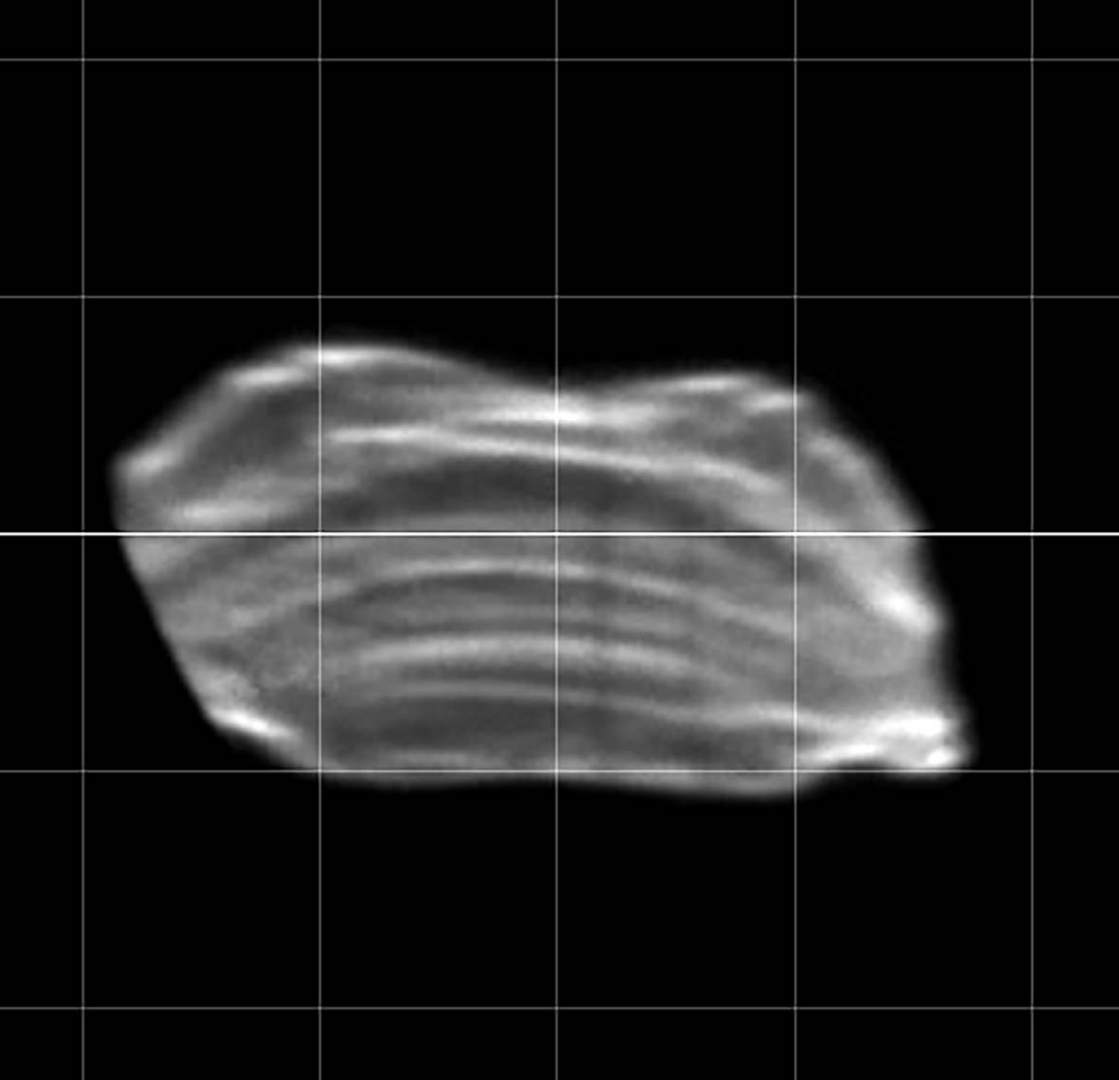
Image of a phosphor screen placed at the sample position and illuminated by the incident X-ray beam. The grid interval is 0.5 mm, calibrated by movement of the experimental table.
